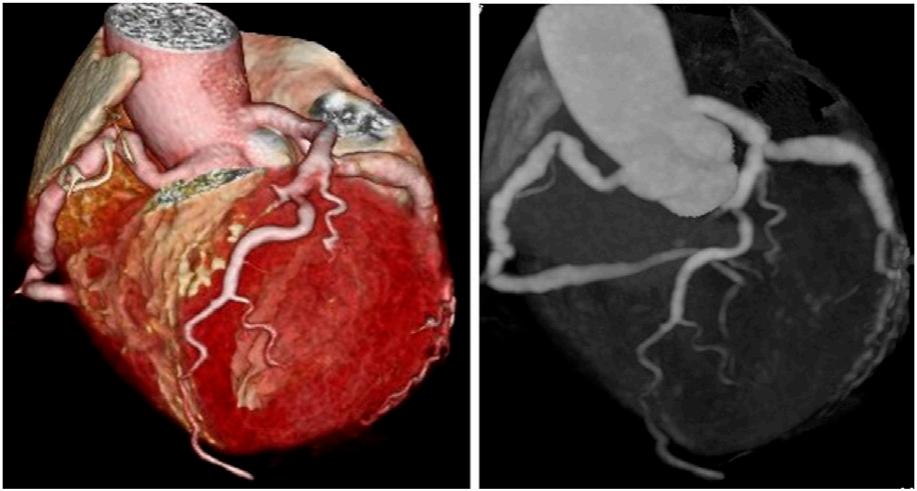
Two types of coronary CT angiography (volume-reproduction model) show diffuse coronary ectasia.
